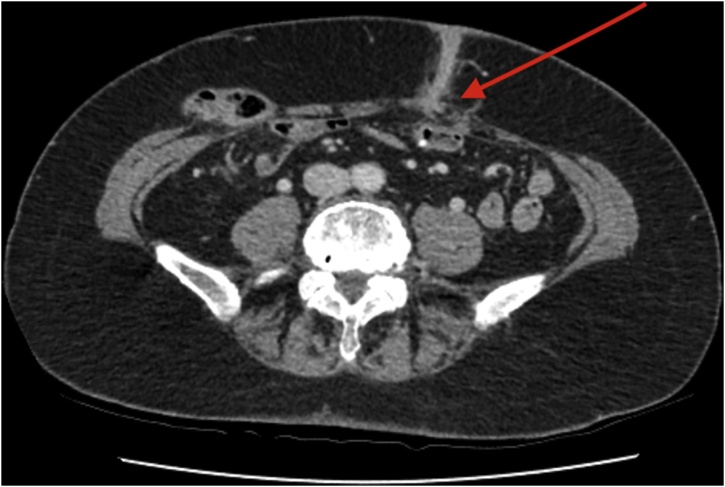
Contrast CT image of the ECF in the axial plane.
